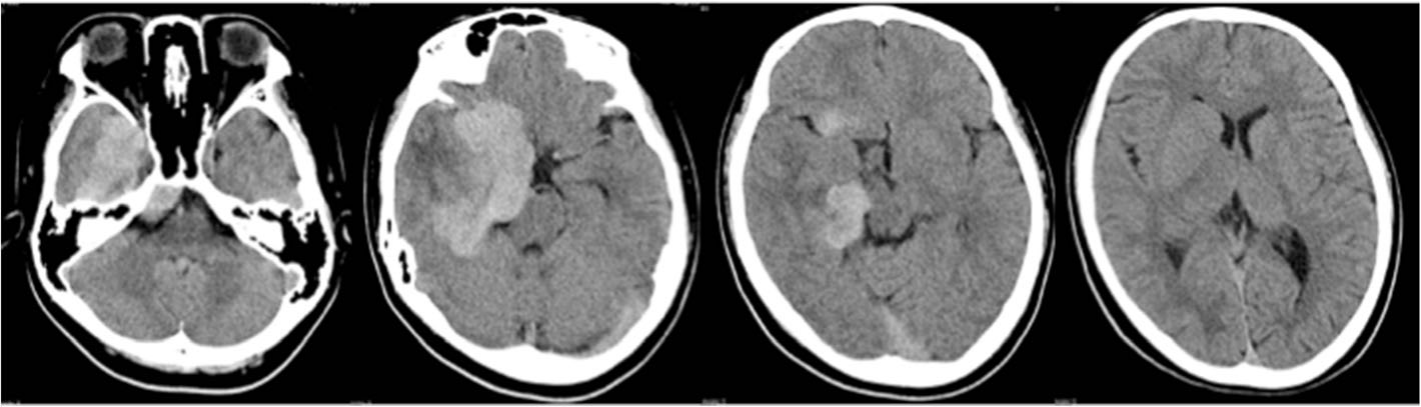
Preoperative plain CT scan of the head showing a slightly high density huge skull base mass lesion on the right side
